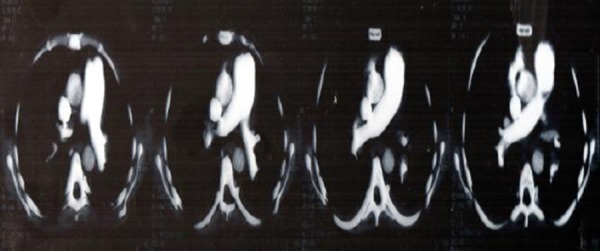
Cardiac CT of the truncus pulmonalis (TP) and principal branches: dilated TP (40mm), dilated RPA (28mm), thrombotic material in TP, massive occlusive thrombus in RPA, and multiple thrombi in secondary branches*.
